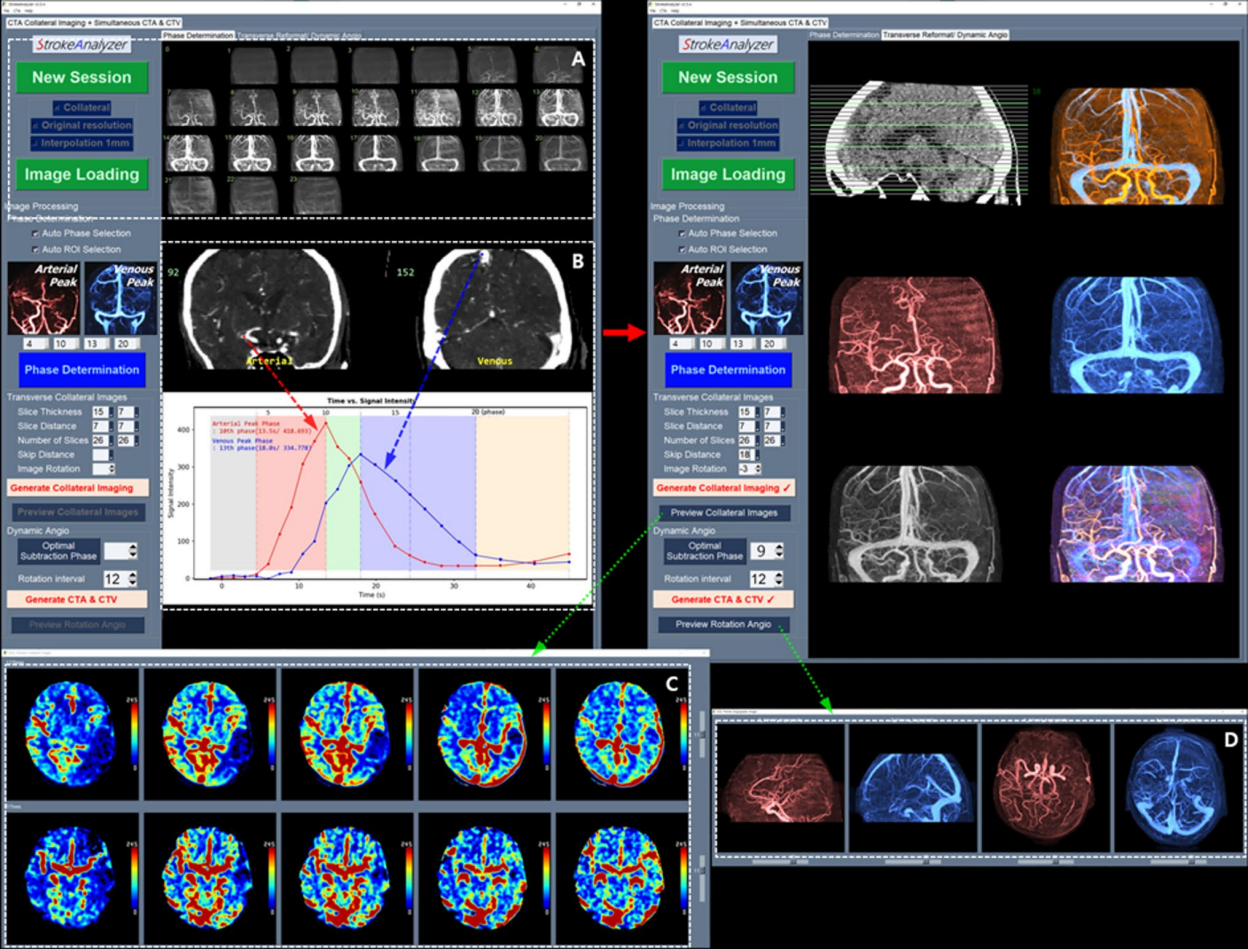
Postprocessing sequences for the CT collateral map using CT perfusion source images: (1) opening and reading DICOM source images of CT perfusion; (2) storing all acquired CT perfusion image data in a 4-dimensional matrix; (3) creating maximum intensity projection images for each time point representing bolus passage for each phase (A); (4) obtaining arterial and venous time-intensity curves for regions of interest on the major arteries (the middle cerebral artery, anterior cerebral artery or terminal internal carotid artery) in the nonischemic hemisphere and the superior sagittal sinus by plotting intensity changes in the region of interest (B); (5) separating the 4-dimensional images from CT perfusion into 5-phase data sets based on bolus passage status, plotted on the time-intensity curves (arterial phase = from the beginning of arrival of the contrast in the major artery to the arterial bolus peak; capillary phase = from just past the arterial bolus peak to just before the venous bolus peak in the superior sagittal sinus; early venous phase = first half of the venous phase from the venous bolus peak to the starting point of the venous plateau; late venous phase = second half of the venous phase; delay phase = from just past the venous phase to the end of the dynamic phase); and (6) reformatting the image data sets of each 5 phases into 5-phase axial image sets (CT collateral map) by averaging the intensities with the desired reconstruction parameters (slice range covering nearly the entire brain, slice thickness ranging from 7 to 15 mm, slice distance, and number of slices) (C). Using the optimal signal difference phase (the time point at which the maximum signal difference between the arterial and venous signal intensity-time curves in the arterial phase), color-coded arteriogram, venogram and dynamic angiography were simultaneously generated (D). The postprocessing time from loading source images of CT perfusion to generating the CT collateral map and angiographies was approximately 5 min
